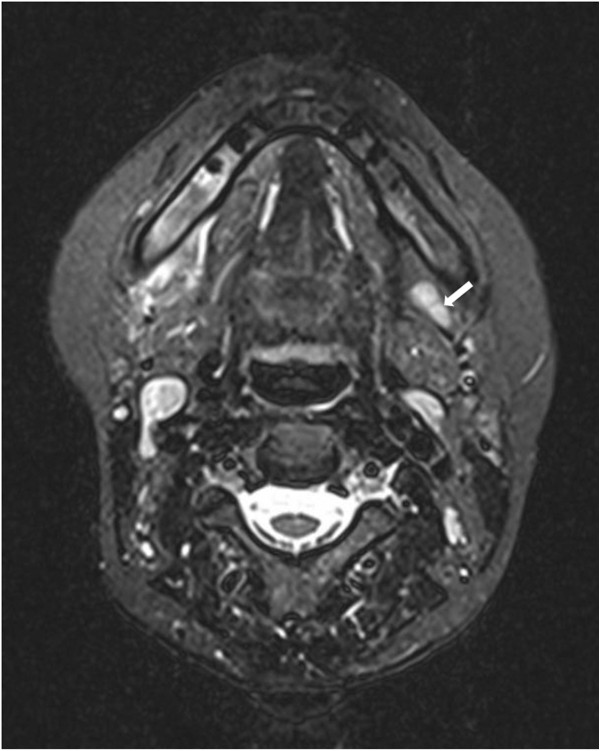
Magnetic Resonance Imaging (MRI), t2 weighted, showing increased signalling in the jaw within the region of teeth 44 until 47 as well as in the ipsi- and contralateral lymph nodes (left side with white arrow). Additionally, perimandibular soft tissue swelling is detectable on the affected side.
